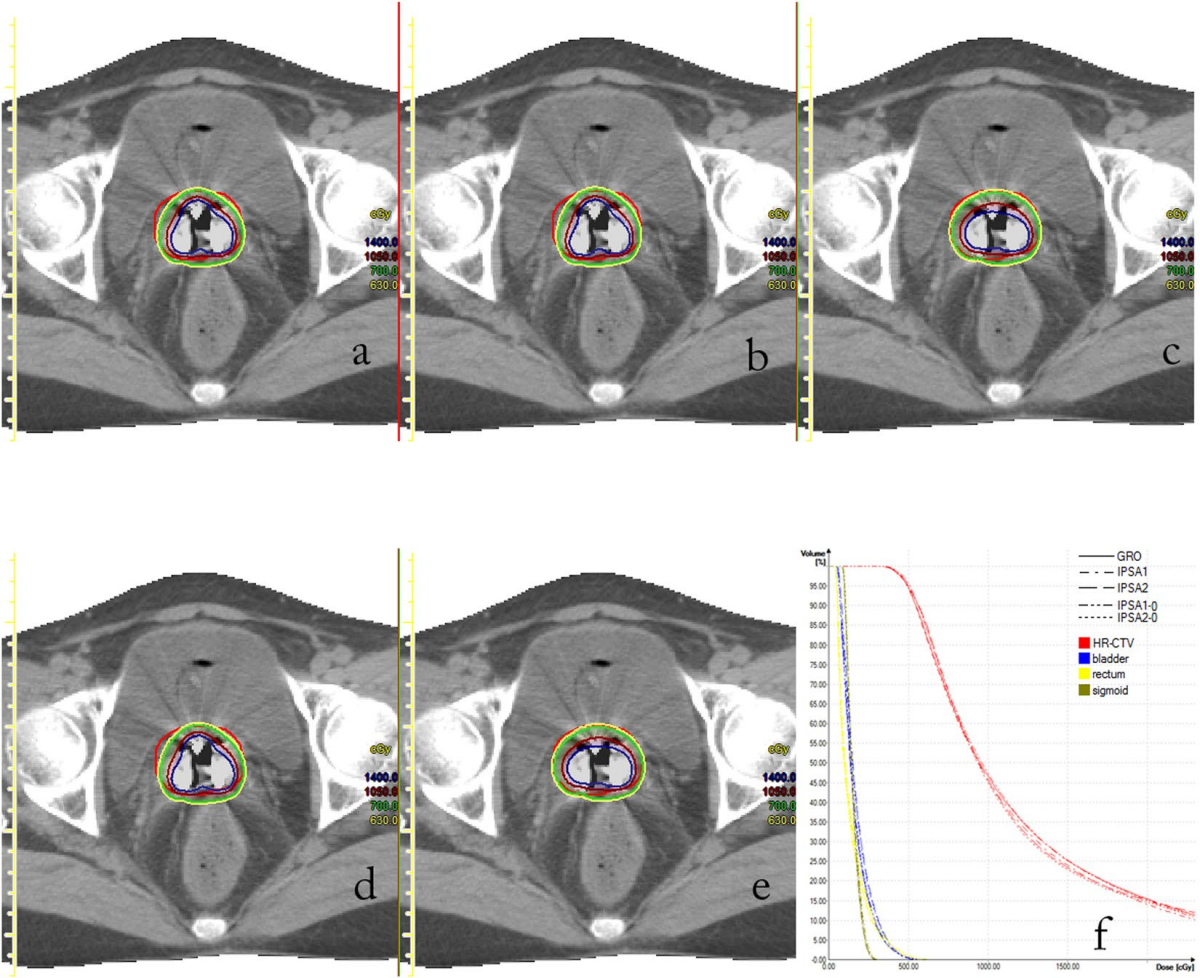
Example of transverse computed tomography images and dose-volume histogram from one patient for brachytherapy planned using (a) GrO, (b) IPSA1, (c) IPSA2, (d) IPSA1-0, or (e) IPSA2-0. (f) The dose-volume histogram.
